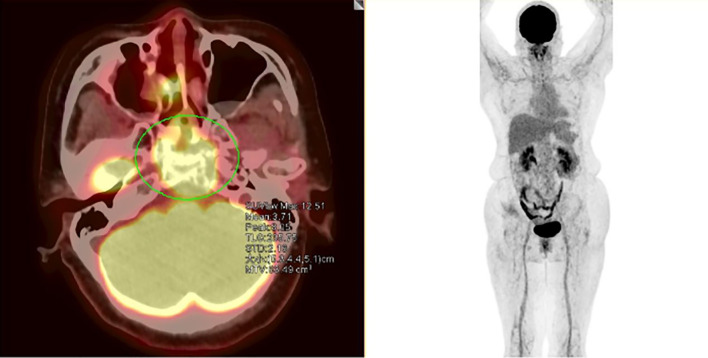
Postoperative PET/CT. PET/CT findings: Increased FDG uptake (SUVmax=12.51) in the sellar region with sphenoid/clival destruction.
